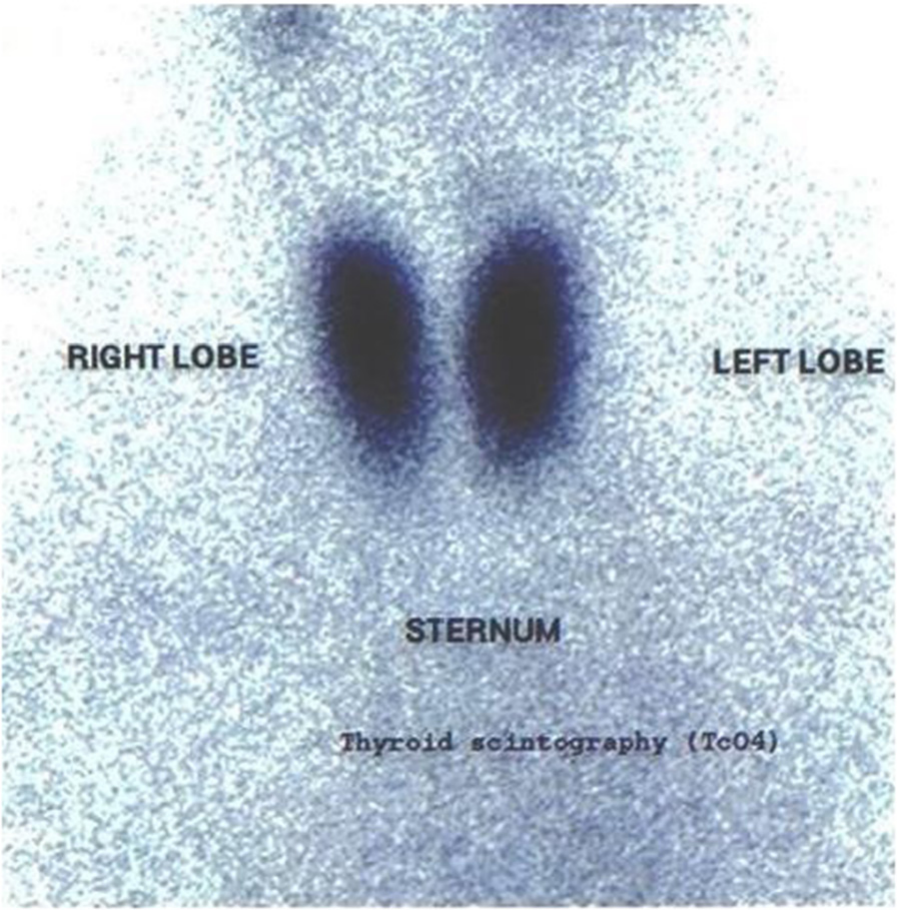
Thyroid scintigraphy with 5 mCi TcO_
